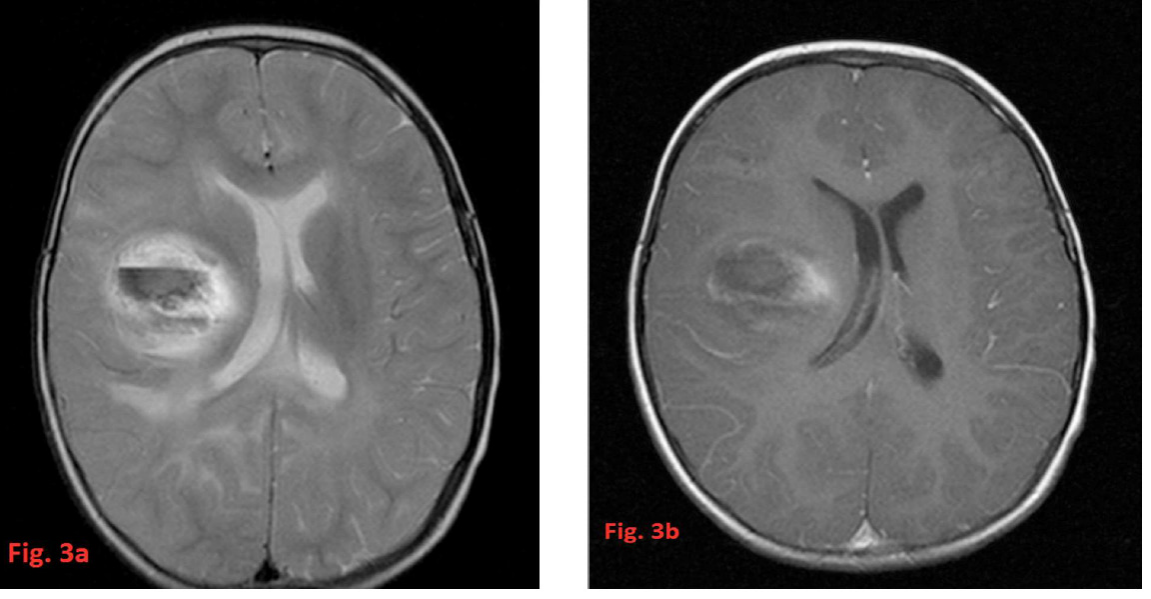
T2-weighted axial image (a) showing a central hemorrhagic part (with a fluid level) of a thalamic glioblastoma together with an increased T2-signal in the posterior corpus callosum, the right frontal region and the left frontobasal parts (not shown) of the brain as a result of multifocal growth. The T1-weighted image at the corresponding level after contrast (b) shows only slight enhancement surrounding the central deoxyhemoglobin core of this tumor part and very ill defined borders.
